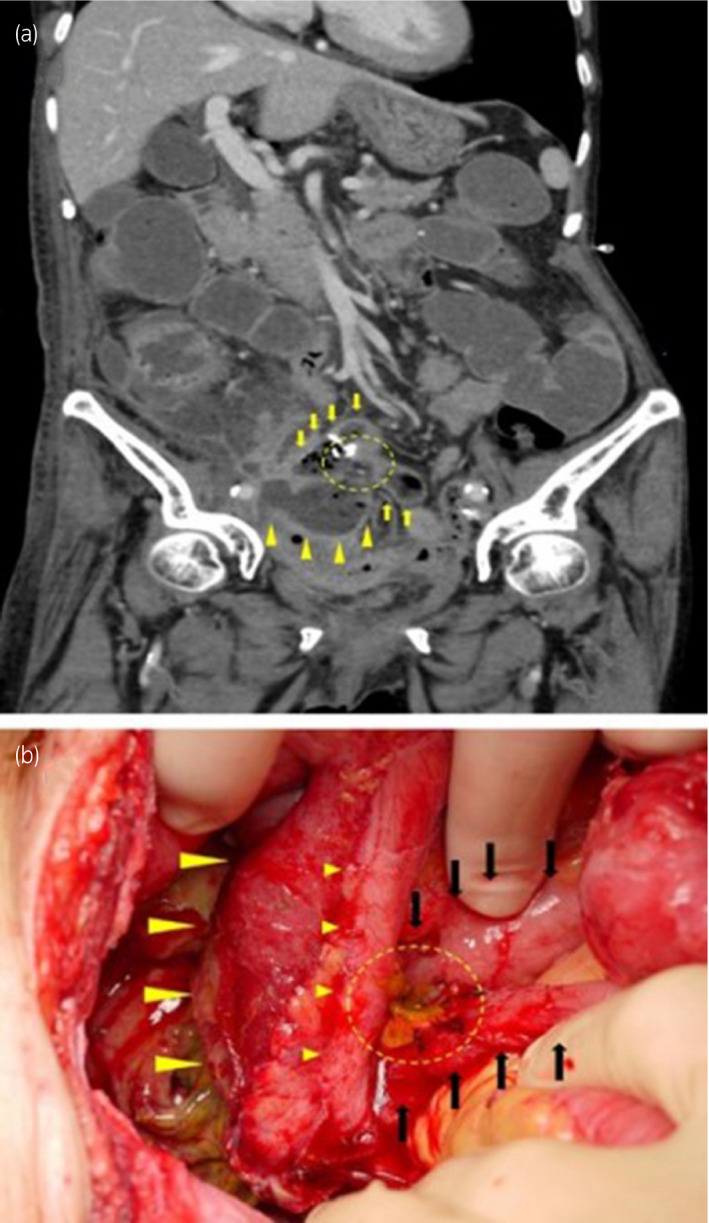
Computed tomography showing anastomotic leakage and an intraperitoneal abscess following a fistula of the ileal conduit, and intraoperative visualization of the anastomotic leakage seen. (a) The yellow arrowheads and the yellow arrows indicate the ileal conduit and anastomosed stumps of the resected small bowel, respectively. The yellow circle shows anastomotic leakage. (b) The big yellow arrowheads and the small yellow arrowheads indicate the ileal conduit and ureter, respectively. The black arrows indicate the anastomosed stumps of the resected small bowel. Anastomotic leakage and stool leakage were recognized (yellow circle).
